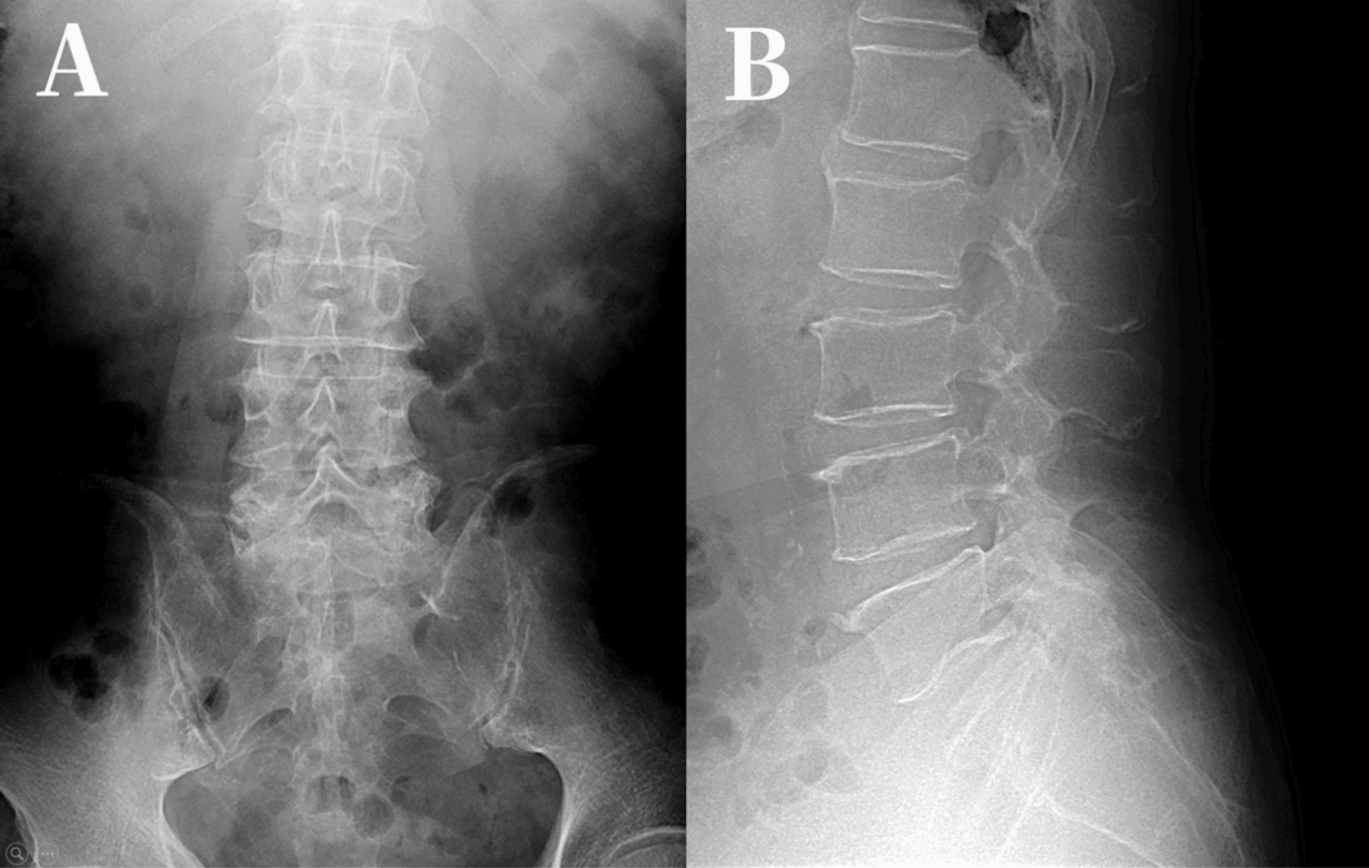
Anteroposterior and Lateral images. (A) Anteroposterior (B) Lateral radiographs showing no apparent fracture (10 days after injury).
